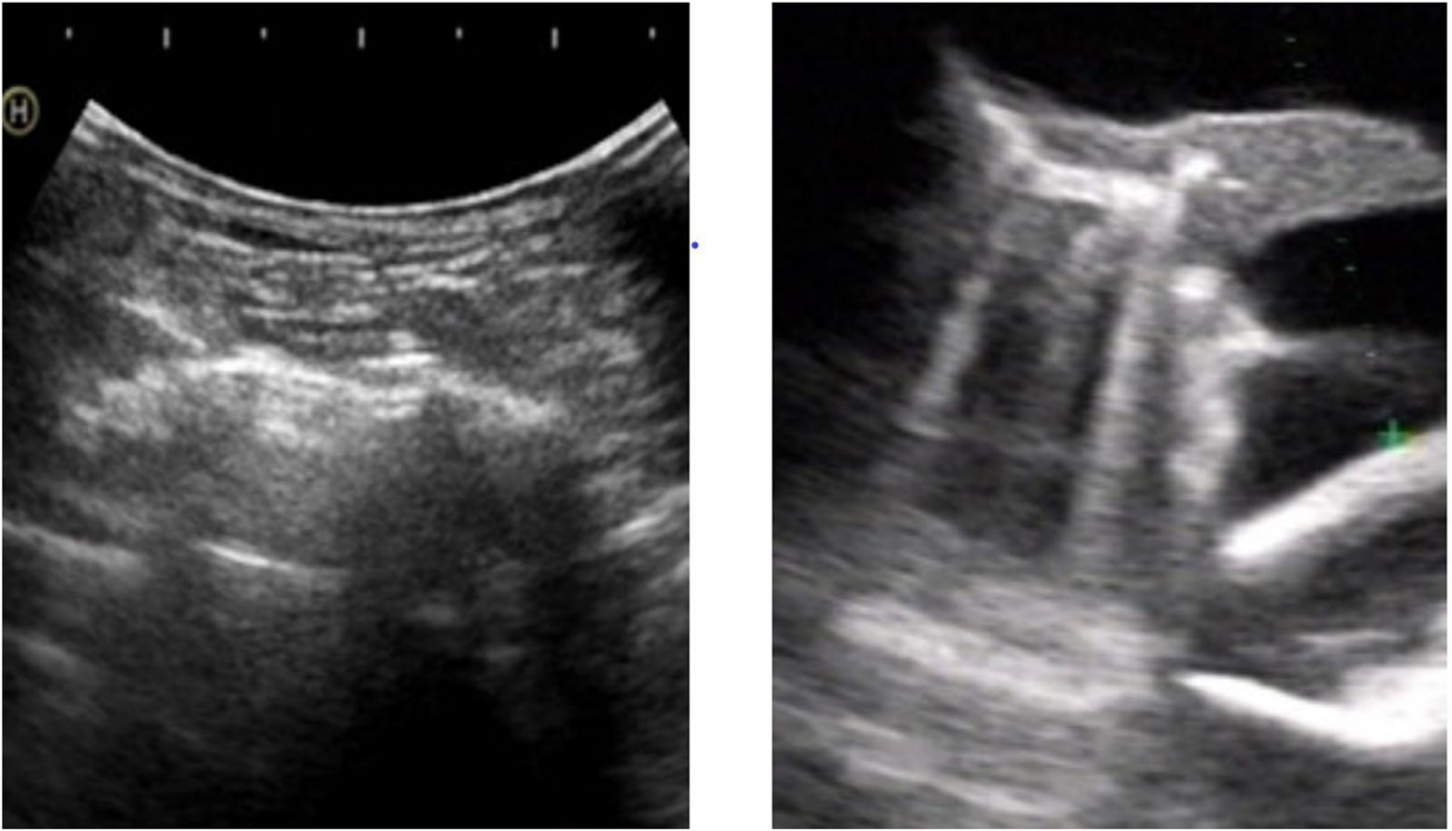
The ultrasonography of normal lung and pulmonary congestion. (Left) Normal lung ultrasonography: the regular, parallel, horizontal hyperechogenic lines are due to the lung-wall interface. (Right) Pulmonary congestion: lung comets are hyperechogenic, coherent vertical bundles with narrow basis that extends from the transducer to the further border of the screen.
